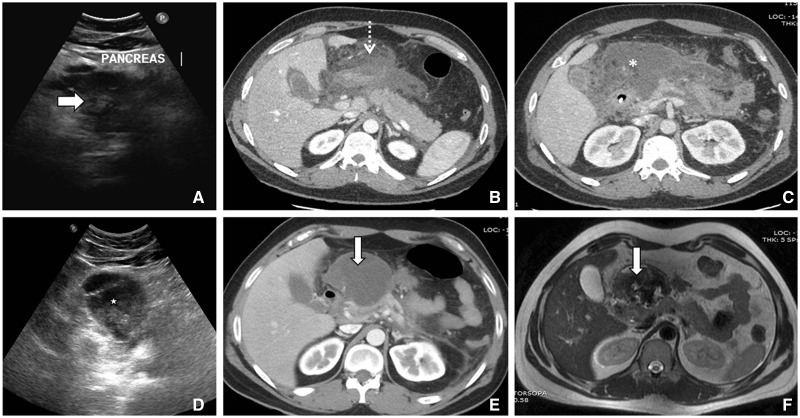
Serial images of a 26-year-old male presenting with acute necrotizing pancreatitis. (A) First ultrasound showing bulky heteroechoic pancreatic (arrow) echotexture. (B) Axial contrast-enhanced CT, showing acute necrotic collection (dashed arrow) with absence of a well-defined wall involving predominantly the peripancreatic region and neck of pancreas. (C) Subsequent axial CT scan around 15 days later, showing increasing heterogeneity (asterix) with areas of ill-defined wall. (D) Ultrasound 4 weeks later than the first ultrasound, showing walled-off necrotic collection (star) in the form of well-defined wall with coarse internal echoes. (E) Axial CT and (F) T2-weighted MR image showing walled-off necrosis (arrow).
